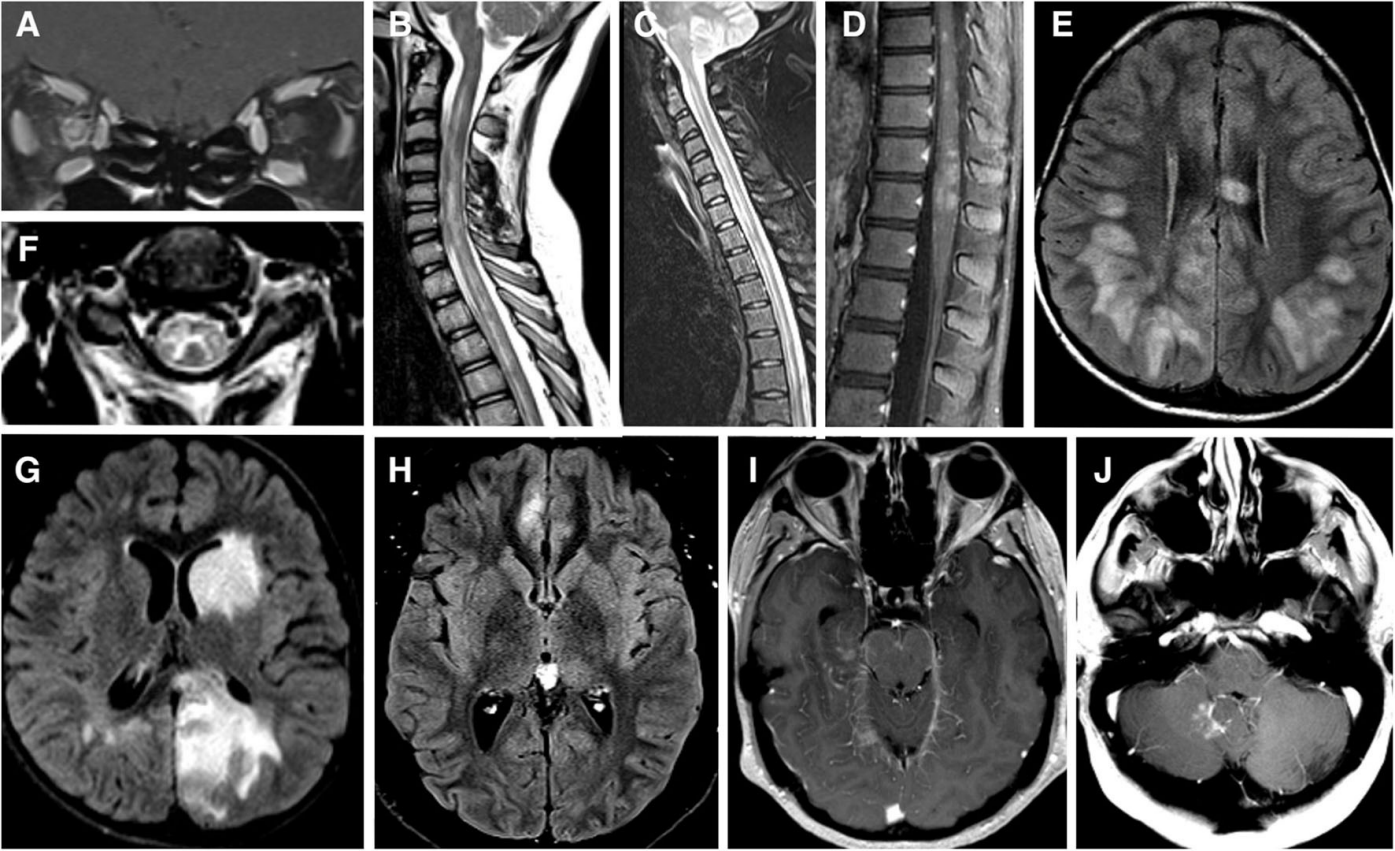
(A) MRI brain T1 coronal post gadolinium contrast showing contrast enhancement of bilateral optic nerves and right optic nerve sheath consistent with perioptic neuritis. (B) MRI spine sagittal STIR showing longitudinal extensive patchy lesion spaning from cervical to thoracic cord. (C) MRI spine sagittal T2 showing hyperintense longitudinally extensive “pseudo-dilation” of central canal. (D) MRI spine sagittal T1 post gadolinium contrast showing patchy enhancement of the conus medullaris. (E) MRI brain axial FLAIR showing large subcortical and septal white matter lesions in a pediatric patient presenting with ADEM. (F) MRI brain axial T2 with hyperintense “H” sign outlining the central gray matter of the upper cervical cord in a teenager with myelitis. (G) MRI brain axial T2 with “fluffy” hyperintense lesion of gray and white matter of the left caudate and left occipital parietal regions in a pediatric patient who presenting with ADEM. (H) MRI brain axial T2 showing unilateral FLAIR hyperintensity and edema of right mesial frontal cortex in a patient with FLAMES syndrome. (I) MRI brain axial T1 post gadolinium contrast showing leptomeningeal enhancement of the midrain and right mesial temporal lobe. (J) MRI brain axial T1 post gadolinium contrast showing a lesion adjacent to the cerebellar vermis and dorsal medulla in a patient with brainstem syndrome and no other lesions.
